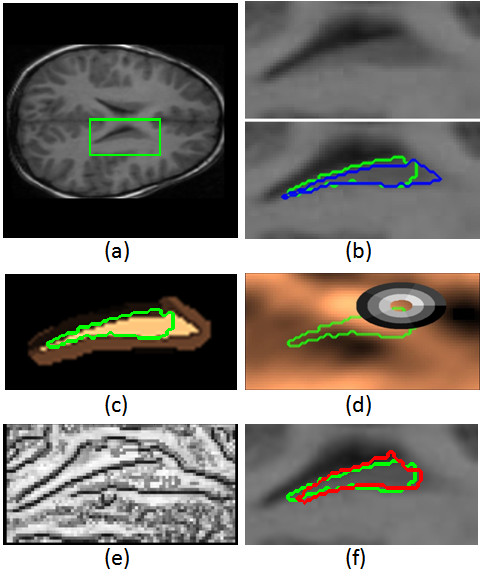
Framework steps. (a) Original MRI scan, (b) Top: Crop from the MRI scan, Bottom: Atlas-based segmentation (blue) and GT (green), (c) Unsupervised probability values P_u_(*L*_*p *_= "cau") and GT (green), (d) Supervised probability values P_s_(*L*_*p *_= "cau") and GT (green), (e) Boundary potentials *B*(*L*), (f) Image crop, GT (green) and CaudateCut result (red).
